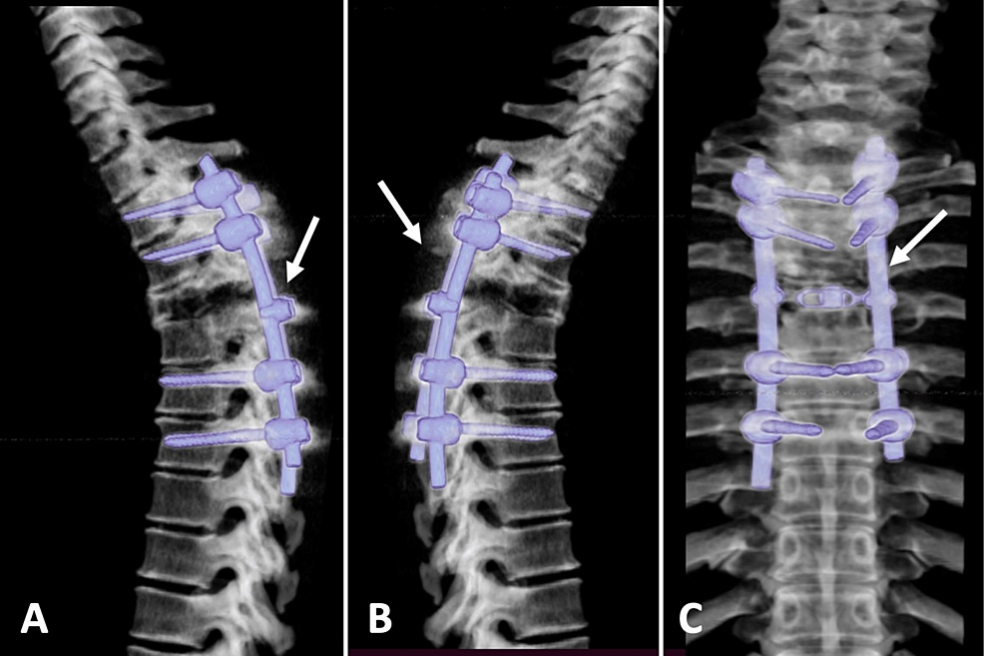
CT with post-surgical 3D reconstruction Lateral (A and B) and anteroposterior (C) views show adequate placement of the fixation system (white arrow).
